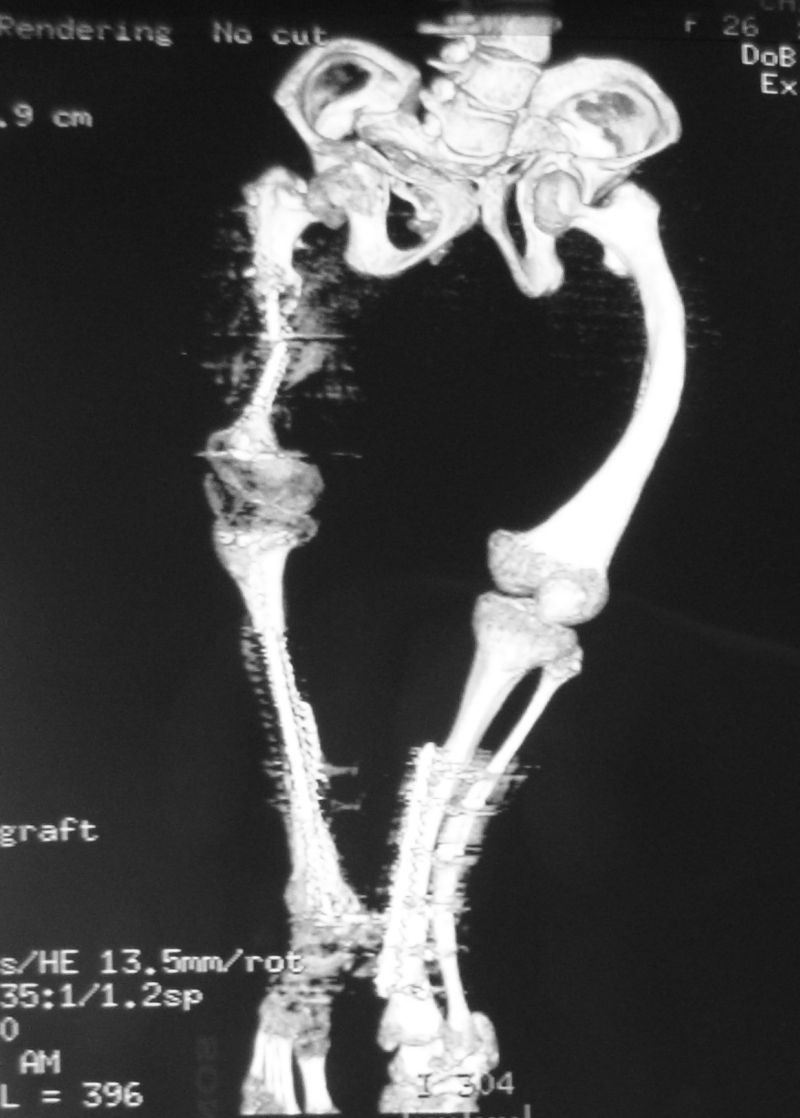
The 3D-CT showed a severe distortion of the proximal right femoral extremity with a hypoplastic configuration and an apparent shorter right femur
